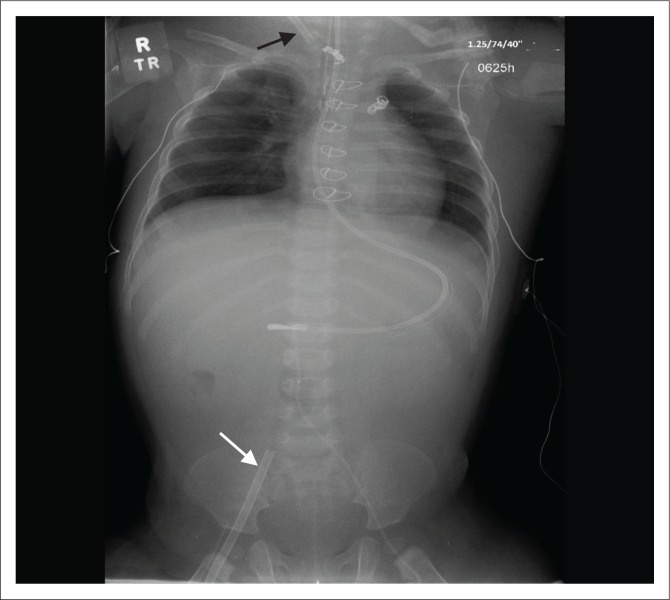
Chest radiograph showing a veno-arterial extracorporeal membrane oxygenation placed in a 1-year-old male child with respiratory failure, with the arterial cannula in the right internal carotid artery (black arrow) and the venous cannula in the right femoral vein (white arrow).
